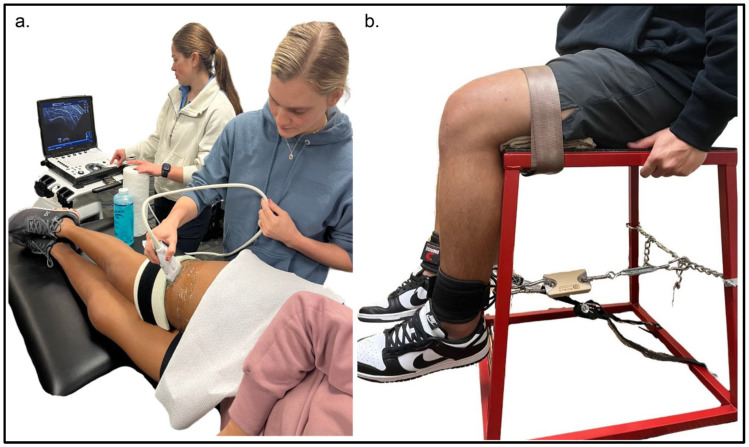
Visual depiction of our methods used to acquire B-mode ultrasound images from the quadriceps (vastus lateralis) (a) and our custom setup used to assess isometric peak torque with a Tindeq dynamometer (b). For strength testing, participants were positioned in a seated posture with the hip and knee at 90° flexion. A strap secured around the distal tibia was connected to the Tindeq dynamometer to measure maximal voluntary isometric contractions of the quadriceps. This configuration enabled consistent and reproducible torque assessment across testing sessions.
